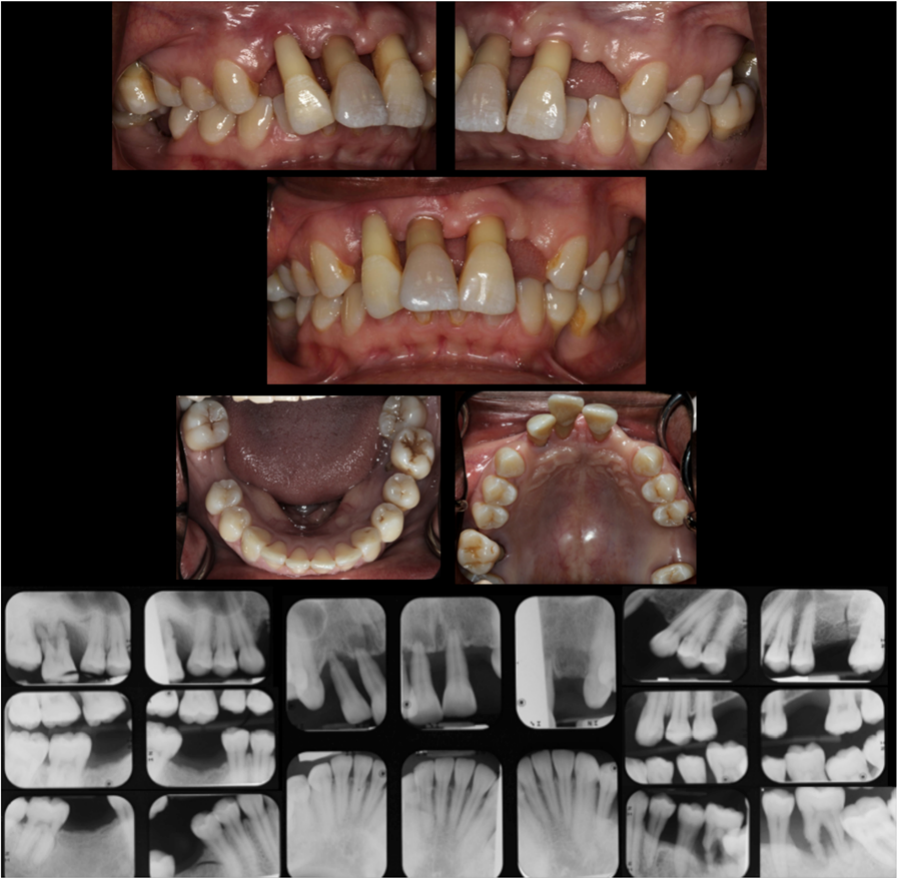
The included figure is sample of a full set of radiographs and intraoral photos provided in the electronic case reports. This particular set is Case 8; A 27 year old female who presented to the NYUCD Periodontics clinic with no significant medical or social history and a chief complaint of spaces between her front teeth
